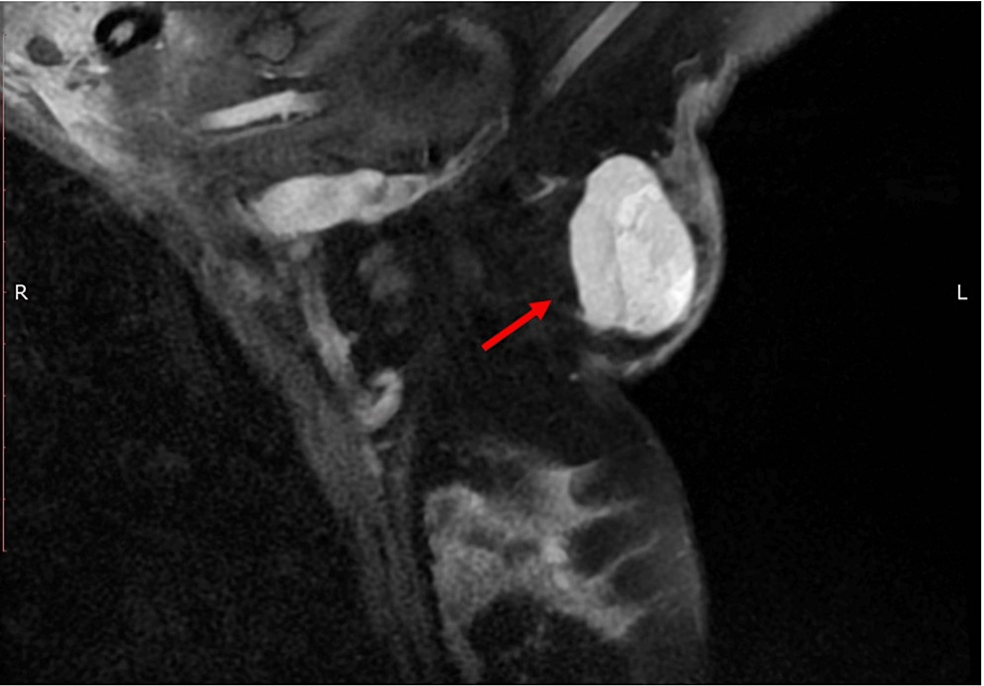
Breast MRI revealed a 4-cm oval mass with microlobulated borders and a type I enhancement curve (red arrow). Non-enhancing internal septations could be seen.
